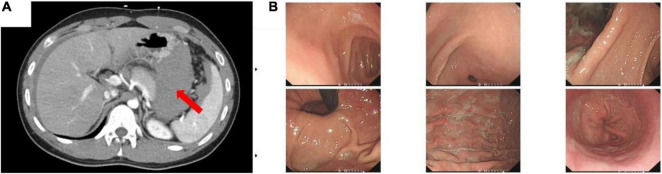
(A) Enhanced computed tomography (CT) of the abdomen. Red arrow showed a 10 × 5.2 cm^2^ high-density shadow in the gastro-pancreatic gap. (B) Gastroscopy showed that the gastric mucosa was smooth and had no ulcer or bleeding spot in the upper digestive tract.
